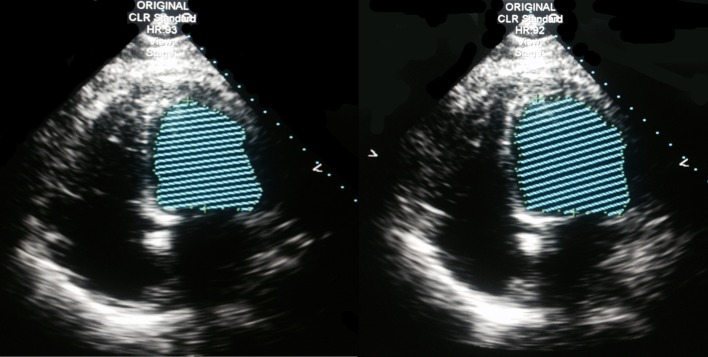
2D echocardiogram, four-chamber view, Simpson method of ejection fraction estimation. Estimated ejection fraction: 15-20%, four-chamber dilatation.
